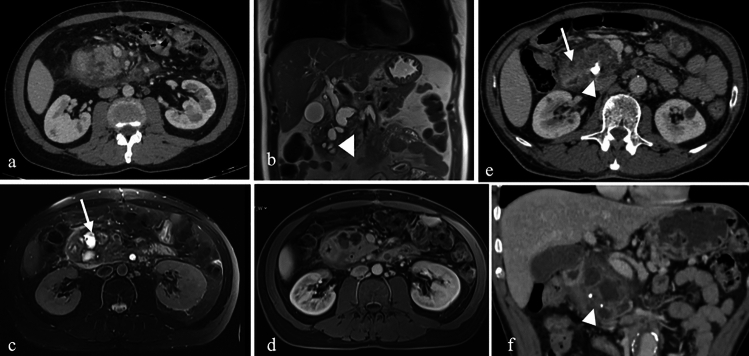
a. Axial IV contrast-enhanced CT, b. Coronal T2-weighted, c. Axial fat-saturated T2-weighted, d. Post-contrast fat-saturated T1-weighted MRI images of a 71-year-old male patient with paraduodenal pancreatitis. Fat stranding and focal fluid collections in the pancreaticoduodenal groove, as well as cystic changes in the duodenal wall, are visible (arrows). Additional findings include dilatation of the common bile duct, choledochus, and pancreatic duct (arrowhead). e. Axial and f. Coronal IV contrast-enhanced CT images of another patient (67-year-old female) with similar findings, along with calcifications within the cystic changes (arrowhead)
